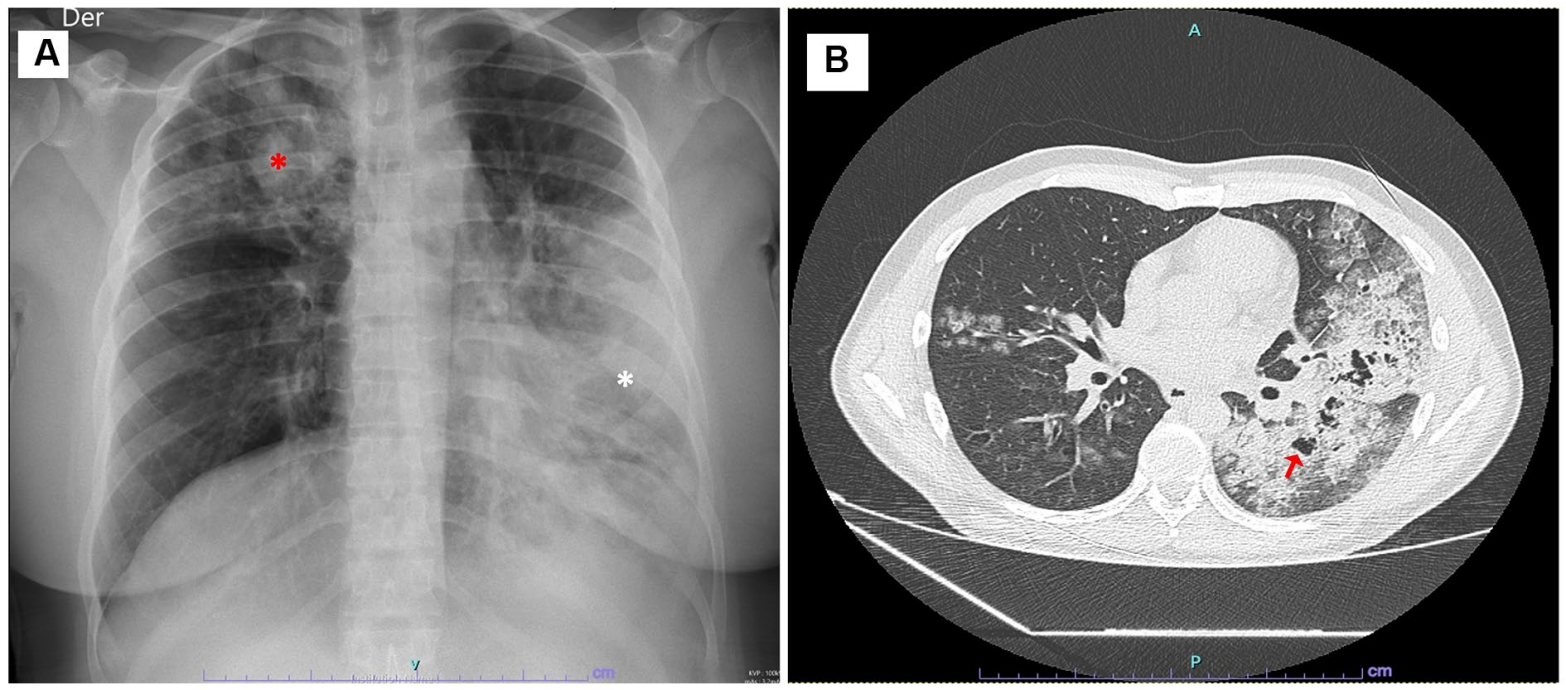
(A) Chest X-ray image revealing a consolidative opacity throughout the entire left lower lobe (white asterisk) and multiple alveolar filling opacities in the upper right lobe (red asterisk). (B) Chest computed tomography (CT) scan revealing consolidated and ground-glass opacities in the left lower lobe with the presence of pneumatoceles (red arrow), consistent with necrotizing pneumonia.
